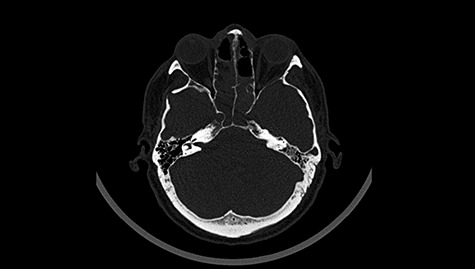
Head CT after polypectomy—seen defects of the upper left orbital wall. (Department of Neurosurgery own material).
